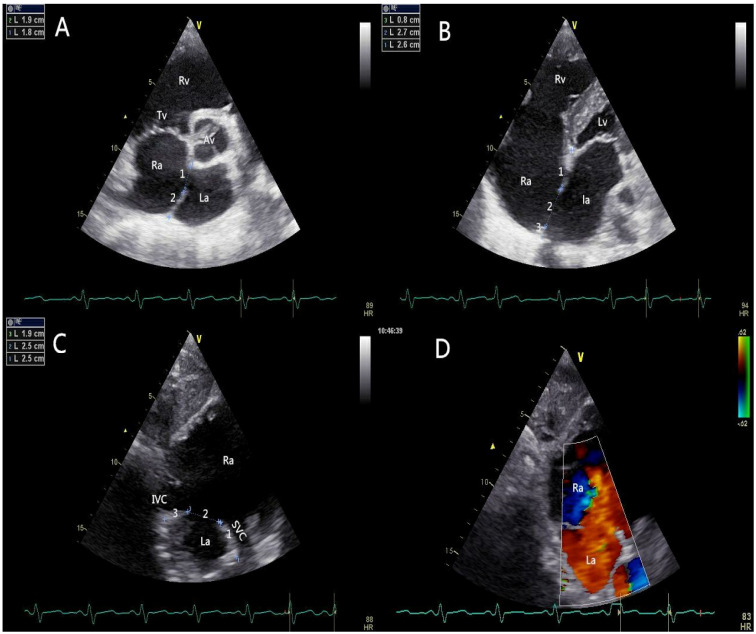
Pre-procedural transthoracic echocardiographic assessment. (A) The short-axis left parasternal view of aortic valve delineated the aortic rim and the posterior rim of the defect; (B) The four-chamber view showed the atrioventricular valve rim and the upper rim; (C) The short axis subcostal view showed the SVC rim and IVC rim; (D) The short axis subcostal view (coloured) showed the blood flow cross the atrial septum (left to right). AV: aortic valve; RA: right atrium; LA: left atrium; RV: right ventricle; LV: left ventricle; TV: tricuspid valve; IVC: inferior vena cava; SVC: superior vena cava.
